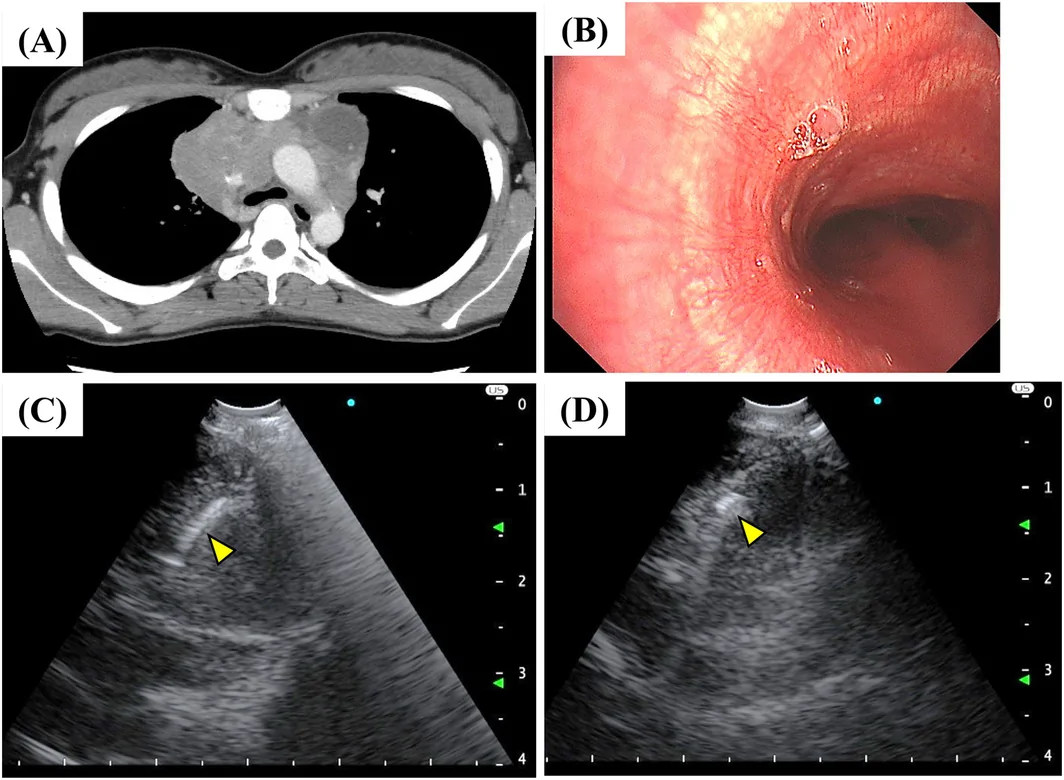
(A) Contrast‐enhanced chest computed tomography showing an anterior mediastinal mass compressing the trachea and carina. (B) Bronchoscopic findings demonstrate narrowing of the tracheal lumen due to extrinsic compression. (C) Endobronchial ultrasound (EBUS) images during EBUS‐transbronchial needle aspiration using a 22‐gauge needle (yellow triangle). (D) EBUS images during EBUS‐guided cryobiopsy using a 1.1‐mm cryoprobe (yellow triangle).
